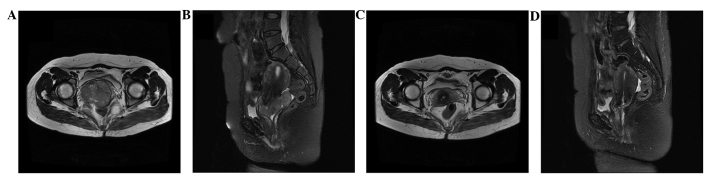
(A and B) Initial pelvic MRI showing a soft-tissue mass on the cervix, with a diameter of 7 cm, which invaded the bilateral parametrial ligaments and extended to the pelvic wall. (C and D) Pelvic MRI following treatment revealing that the cervical tumor had completely disappeared, indicating the efficacy achieved complete remission. MRI, magnetic resonance imaging.
